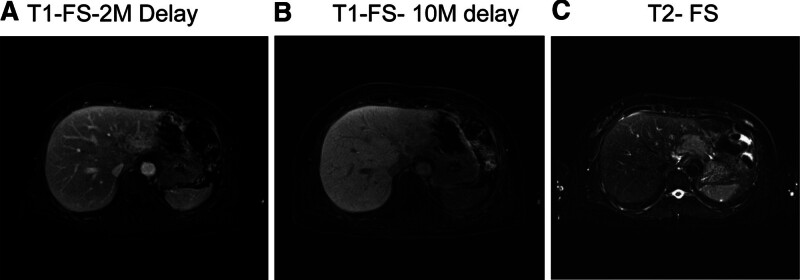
MRI performed 1 mo after the completion of radiation therapy. MRI revealed an irregularly shaped mass in the left lateral segment of the liver exhibiting high signal intensity on T2-weighted images and subtle enhancement. These imaging features suggested the involvement of lymphoma in the left lateral hepatic segment. FS = fat suppressed imaging, M = minutes, MRI = magnetic resonance imaging.
